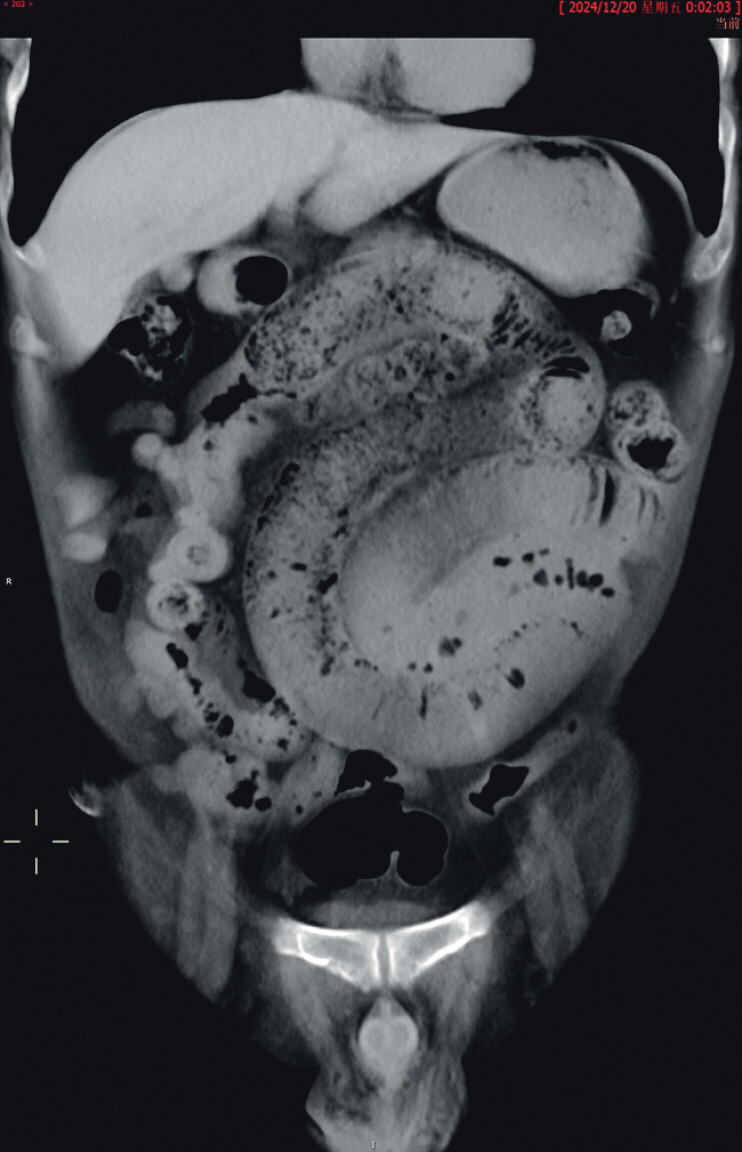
The computed tomography imaging manifestations suggest incomplete intestinal obstruction.
